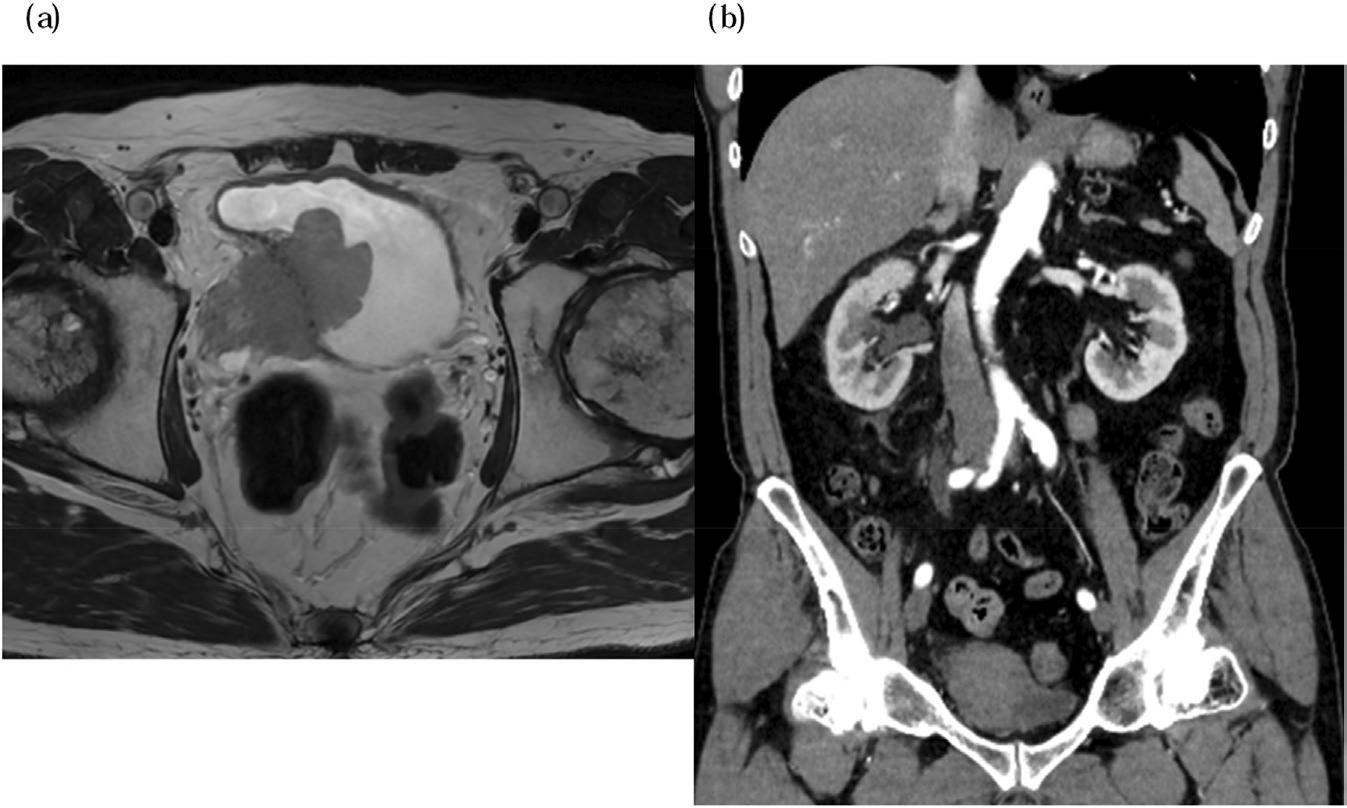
(a) The MRI findings reveal a 5 cm mass located on the right wall of the bladder. There is suspicion of infiltration into the pelvic wall, and infiltration is also noted at the right ureteral orifice. (b) The contrast‐enhanced chest and abdominal CT findings show no evidence of distant metastasis from the bladder tumor. Additionally, the right kidney exhibits hydronephrosis graded as SFU grade 2.
